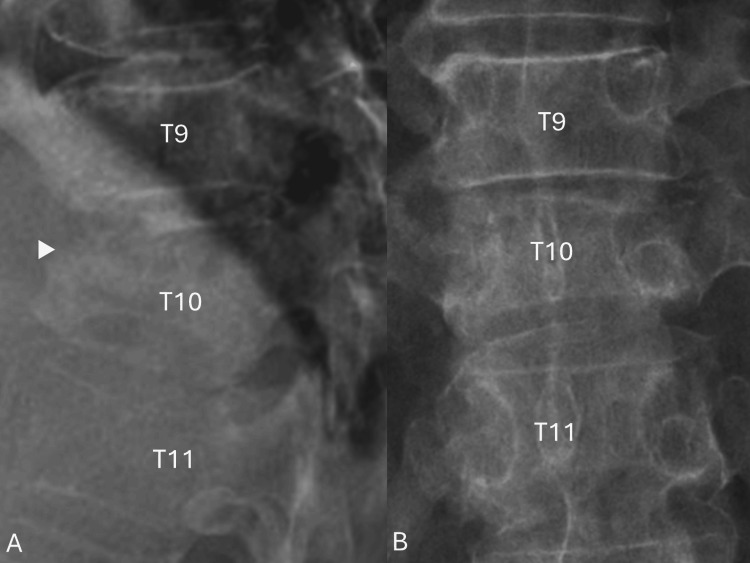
Preoperative plain film of the thoracic spine. (A) Lateral view, anterior wedge fracture, and blurring of the superior end plate of T10 (arrowhead). (B) Anterior-posterior view
